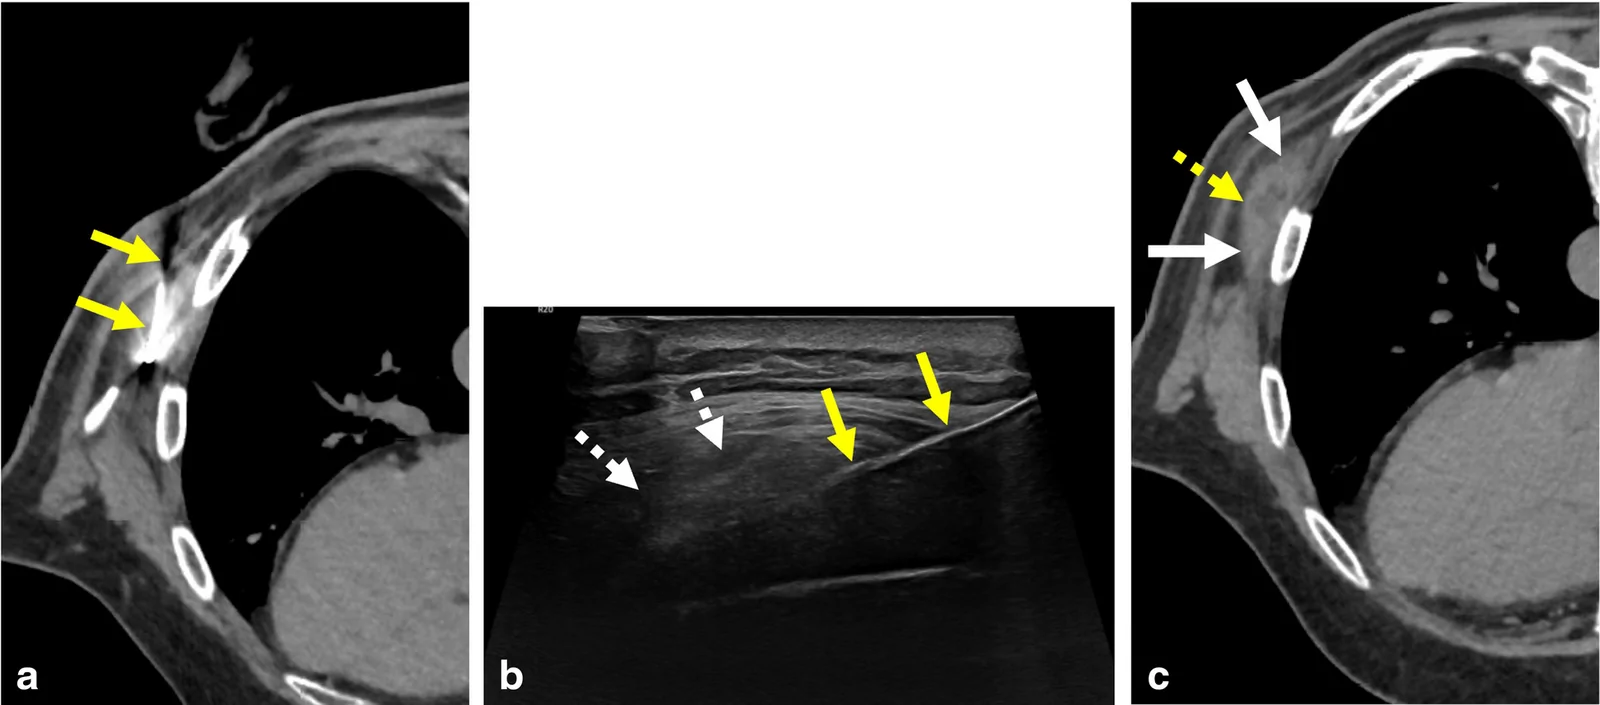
US and CT-guided percutaneous cryoablation of elastofibroma dorsi. a CT and b US image of the cryoapplicator inside the elastofibroma dorsi (yellow arrows). Note the ice ball (dotted white arrows) visible in the US image. c At the end of the procedure, non-contrast CT shows a necrotic area within the lesion (dotted yellow arrow)
